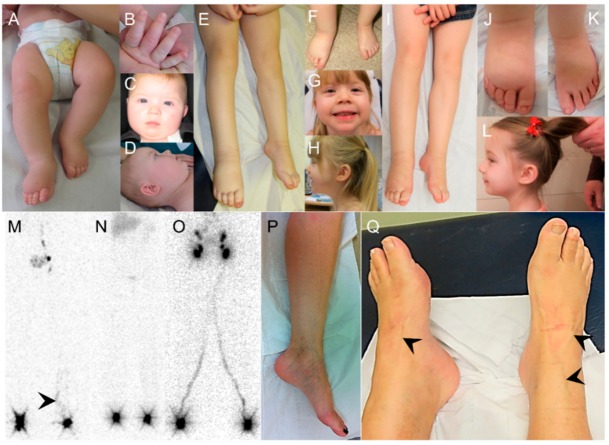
Clinical phenotype of the proband, mother and maternal grandfather and anterior view of lower limb lymphoscintigraphy. (A–D) Proband at her first visit to the genetics clinic at six months. (A) The proband presented as a baby with bilateral lower limb lymphedema. Swelling of the dorsum of the feet was obvious. Deep inter-phalangeal creases and small dysplastic nails were seen. (B) There was mild swelling in the hands. (C) She also had subtle dysmorphism with a round face, high forehead, short neck, hypertelorism, and depressed nasal bridge. (D) There was mild bilateral ear dysplasia and right pre-auricular sinus. (E–H) Proband at two years and 10 months. (E,F) The proband had persistent bilateral lower limb lymphedema of the feet, which extended up the leg to the knees and was more pronounced on the right. (G) Subtle dysmorphic features with a round face, hypertelorism, downslanting palpebral fissures, and depressed nasal bridge. (H) Slightly low, posterior rotated ears with mild ear dysplasia. (I–L) Proband at four years and eight months. (I) Proband presented with persistent bilateral lower limb lymphedema to the knees. (J,K) The swelling was particularly noticeable in the dorsum of the foot, which was more marked in the right foot (J) than the left (K). (L) Slightly low, posterior rotated ears. (M) Lymph scan (anterior view) of proband showing no evidence of uptake of the tracer in the right lower limb lymphatic tracts or inguinal lymph nodes after 3 h (functional aplasia). Uptake in the left lower limb lymphatic vessels remains abnormal and reduced (functional hypoplasia). The left lower leg lymphatic vessels are tortuous in the calf region (arrow head). (N) Lymph scan of a patient with typical Milroy disease with no evidence of main-tract filling nor rerouting (functional aplasia). (O) Lymph scan of healthy control. (P) The mother of the proband (III.1) presented with mild varicose eczema and multiple superficial veins around the ankles. There was also subtle lymphedema on the medial aspects of the ankles. (Q) The maternal grandfather (II.2) had bilateral mild varicose veins. There is noticeable mild lymphedema of the left ankle and varicose veins of both lower limbs (arrow heads).
